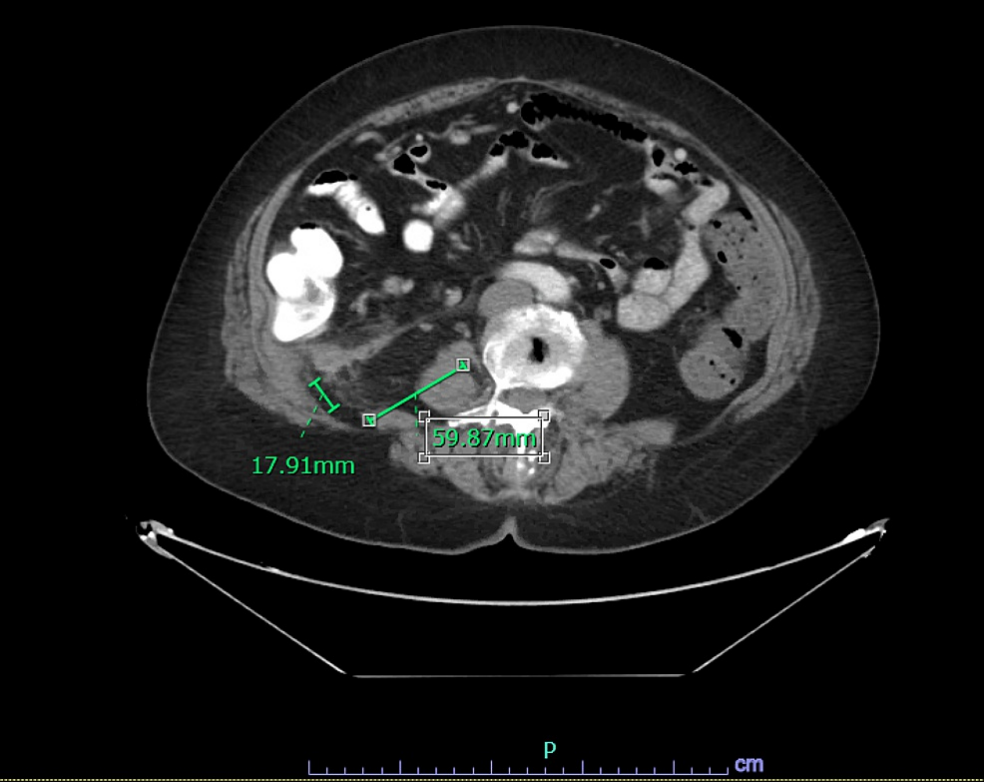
CT chest abdomen and pelvis show abnormal fat stranding adjacent to the hepatic flexure of the colon with retroperitoneal soft tissue mass measuring 5.9 x 1.7 cm in greatest transverse dimensions adjacent to infiltration.
